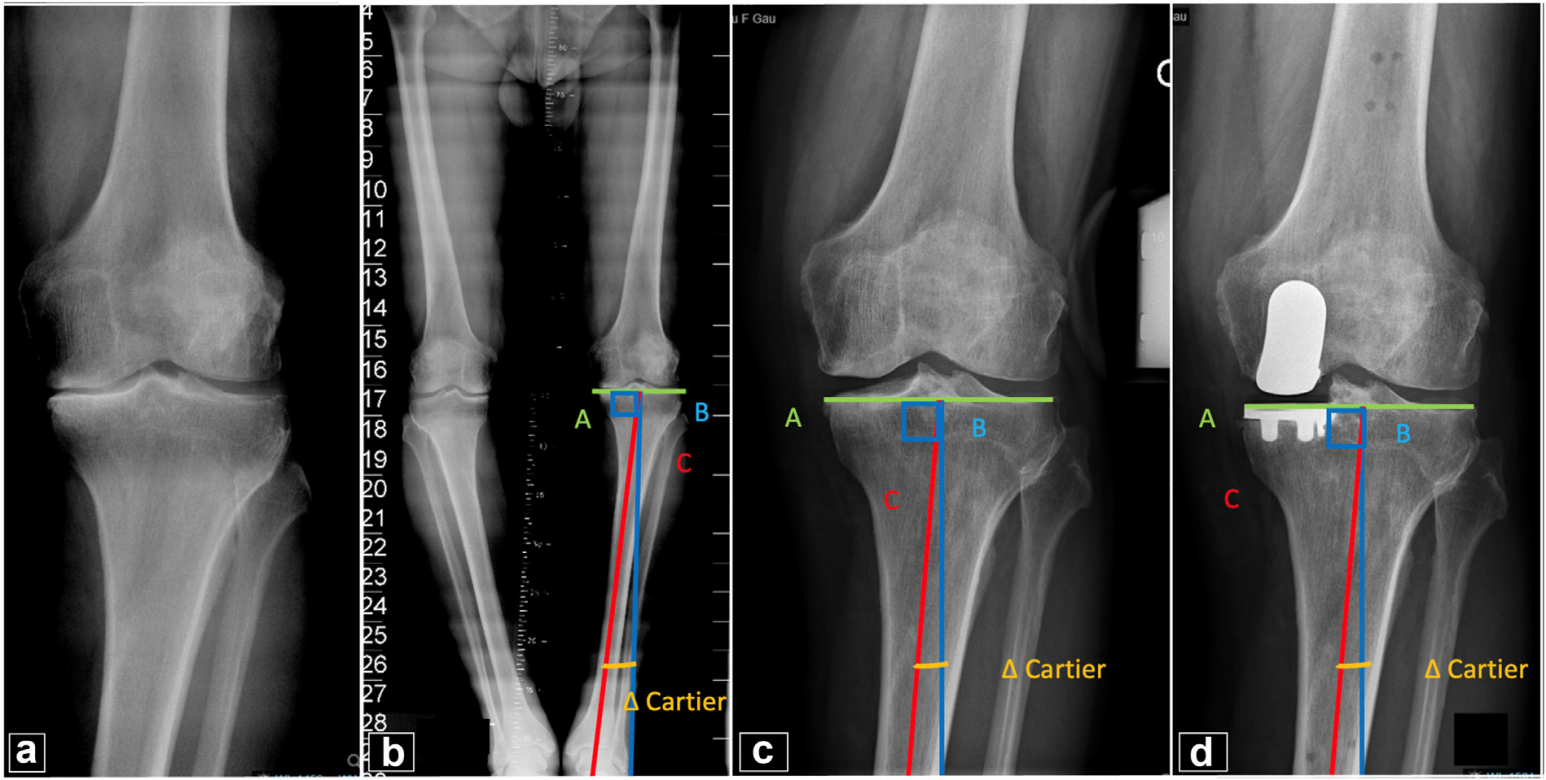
Cartier’s angle. (a) AP radiograph of the left knee showing medial femorotibial osteoarthritis. (b) Long leg x-ray showing how to measure the Cartier’ angle. Line A in green represents the tibial epiphyseal line. Line B, in blue, is perpendicular to line A. Line C, in red, represents the tibial mechanical axis. The angle formed between the line perpendicular to the tibial epiphyseal line (line B) and the mechanical axis of the tibia (line C) is the tibial epiphyseal angle, or Cartier's angle [22]. (c and d) Valgus stress radiograph and postoperative AP radiograph of the left knee showing how the implant has been aligned according to Cartier’s angle [21].
